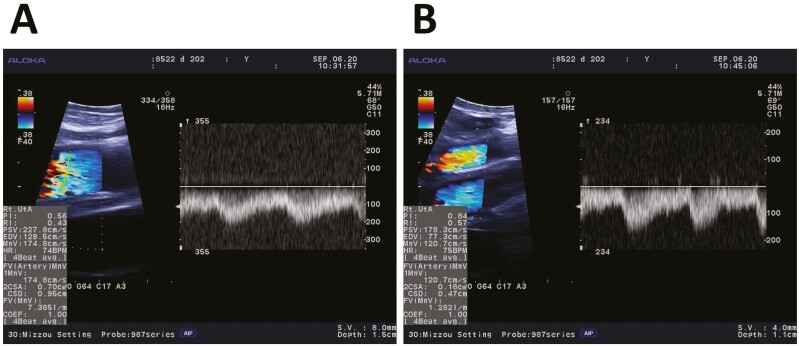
Color Doppler sonograms of the ipsilateral (Panel A) and contralateral (Panel B) uterine arteries of a primiparous beef female on day 202 of gestation. The images were obtained chute side in B|D mode using an Aloka Prosound α6 machine. In B mode (left side), Doppler flow gain was set at 40, the angle of insonation (68° or 69°, respectively) was set parallel to the walls of the artery in a longitudinal view, and the caliper function was used to measure the cross-sectional diameter of the artery perpendicular to the angle of insonation. In D mode (right side), 4 representative and consistent cardiac waveforms were selected (only 3 are shown), from a similar set of frames as those from which the vessel was chosen. The machine calculated all variables, as displayed at the bottom left.
